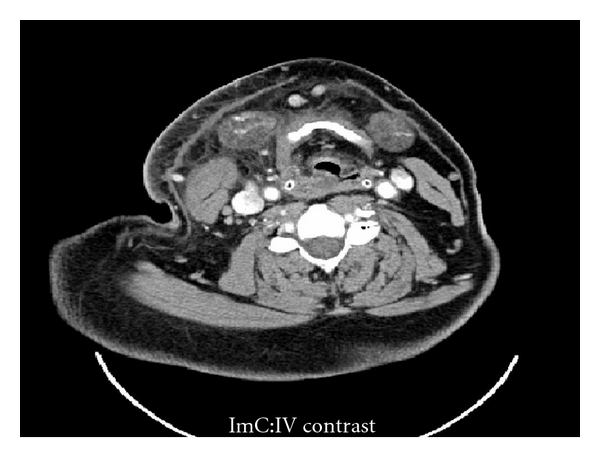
Axial contrast-enhanced CT scan of the patient in Figure 1 showing diffuse swelling of the right submandibular region, stranding of the cervical fat, thickening of the right sternocleidomastoid muscle and the right pyriform fossa. The laryngeal airway is mildly effaced and displaced superiorly.
